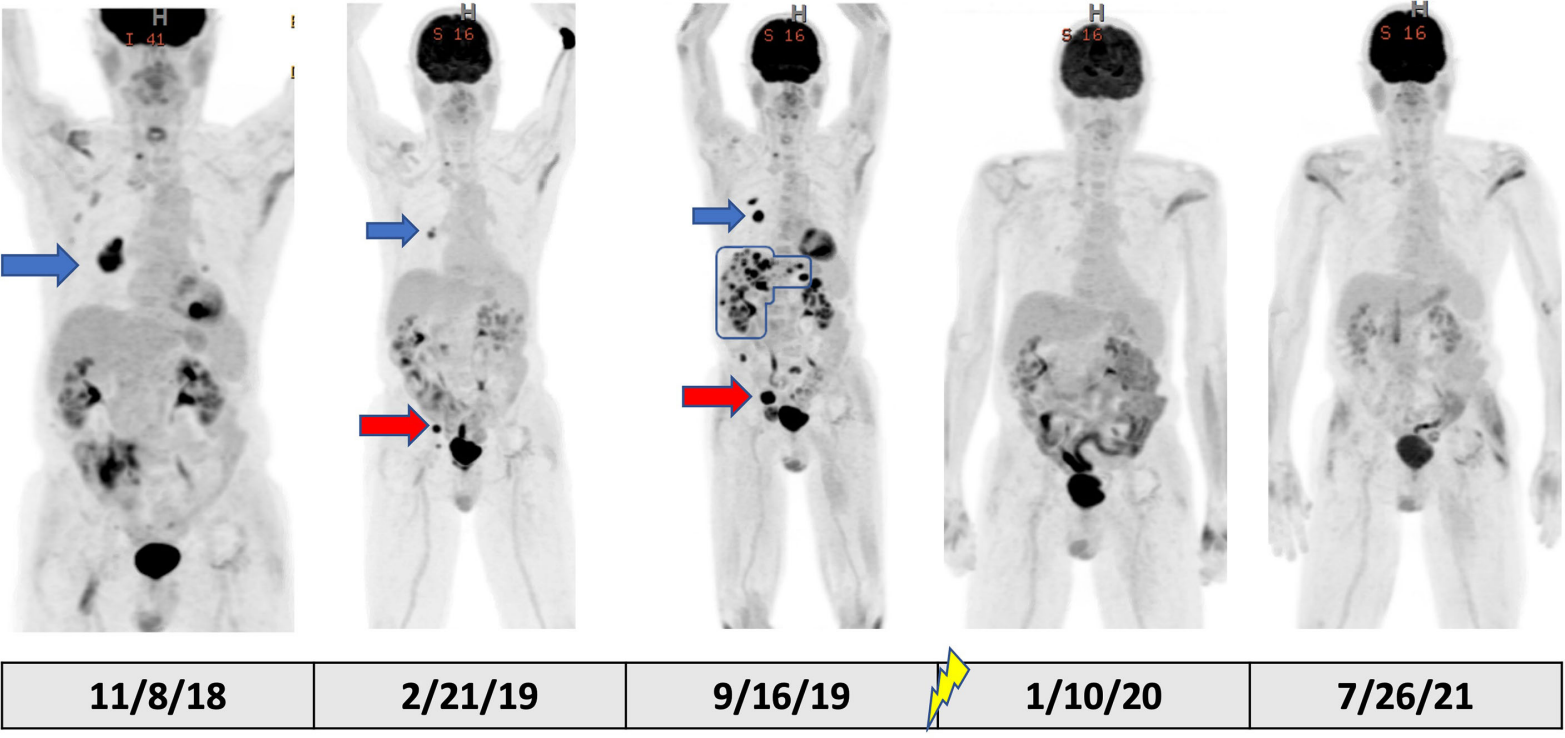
PET/CT scout images over the course of the patient’s treatment. The blue arrows in the figure show the RLL nodule with good response to initial pembrolizumab monotherapy in February 2019 from the baseline scan in November 2018. However, the red arrows demonstrate a site of progression in the right pelvic sidewall through initial pembrolizumab monotherapy representing mixed response to initial pembrolizumab monotherapy. The September 2019 scan, after a break in pembrolizumab therapy for colitis, clearly shows progression of these lesions along with emergency of new, multifocal liver metastases (outlined with blue line). In October 2019, palliative RT was delivered to sacral metastatic lesion (signified by lightning symbol in the timeline). Subsequent PET/CT in January 2020 and July 2021 showed complete and durable response after completion of RT.
